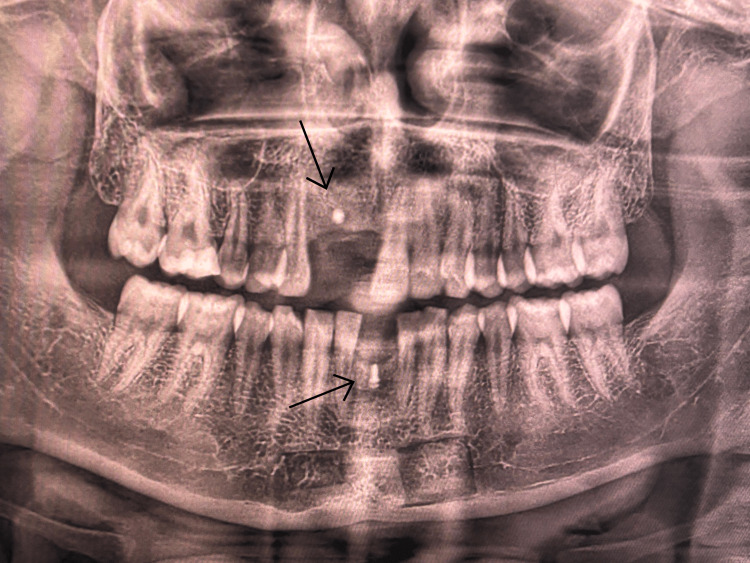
Immediate postoperative orthopantomogram (OPG).
